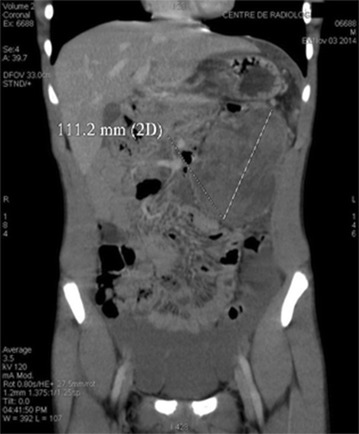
Computed tomography scan showing the mass
